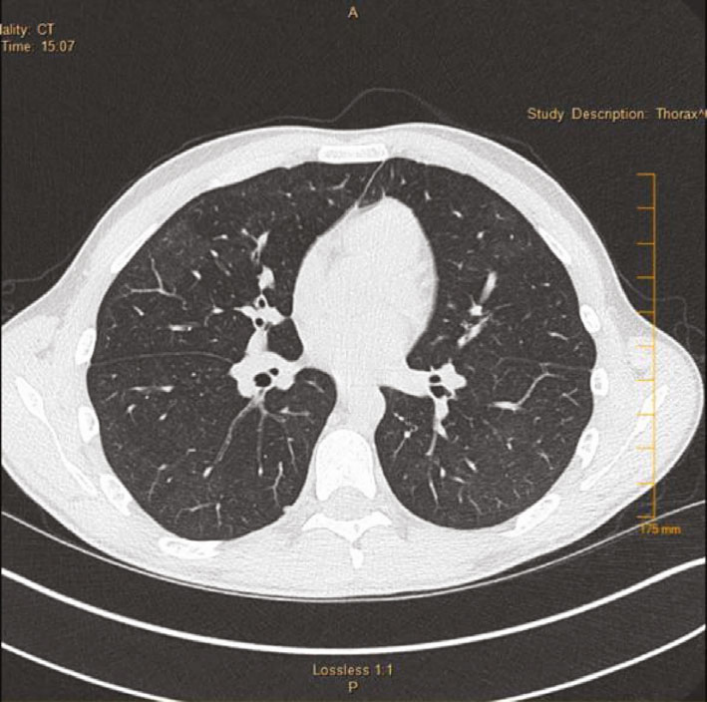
Case 2 chest CT scan 6 weeks post discharge (axial view).
